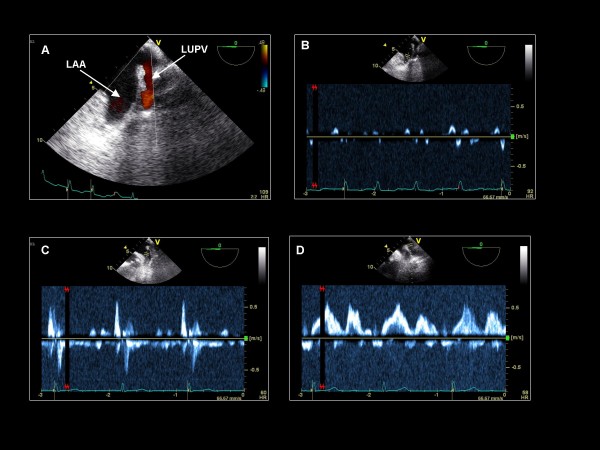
Imaging plane 0°, (A) The left atrial appendage (LAA) and the left upper pulmonary vein (LUPV). Pulse wave Doppler of the LAA demonstrating low emptying velocities in a patient with (B) permanent atrial fibrillation and (C) sinus rhythm. (D) Pulse wave Doppler of the LUPV demonstrating a normal flow pattern.
